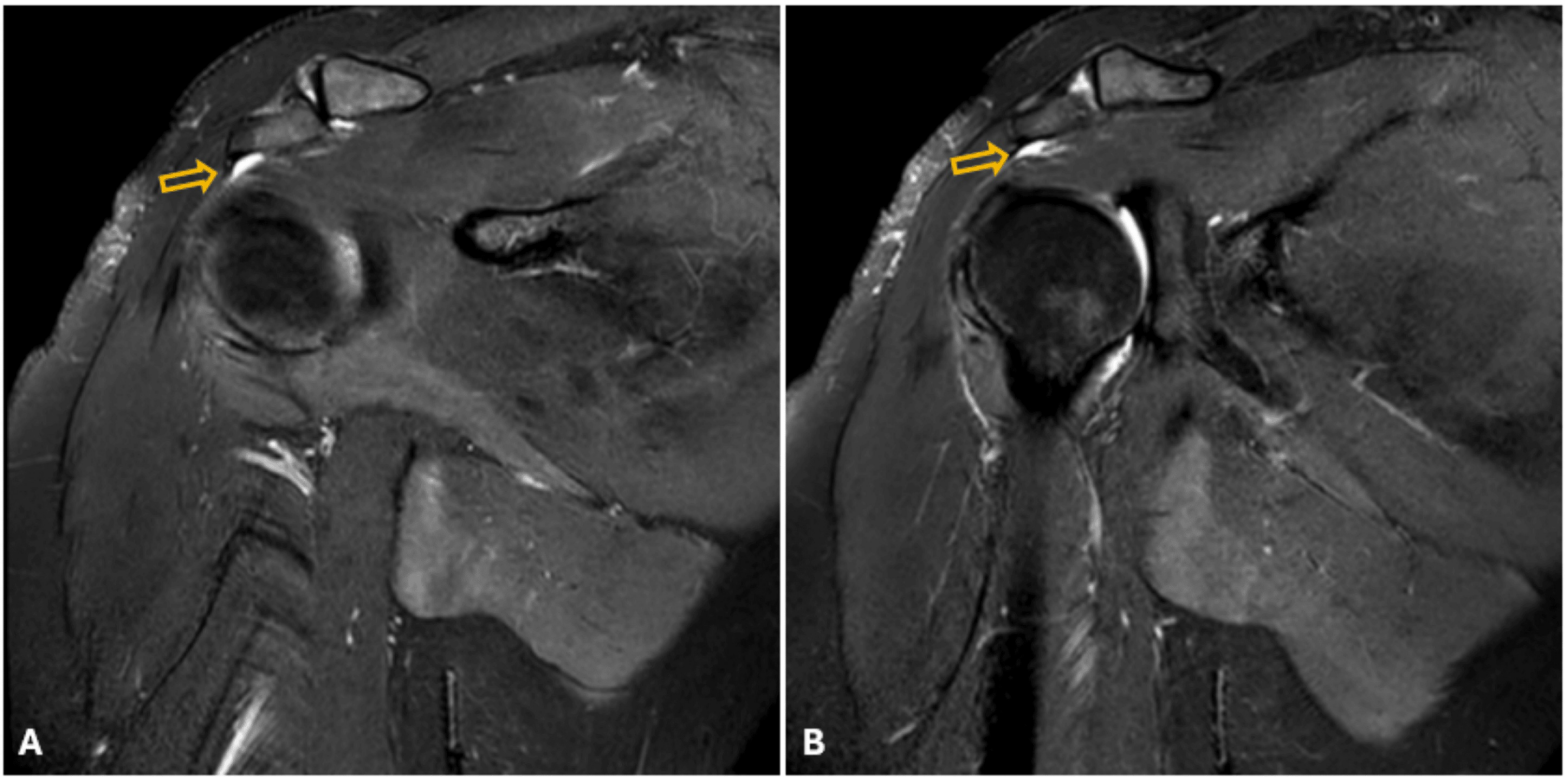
Coronal section magnetic resonance imaging (MRI) images of the right shoulder, indicating suspicion of subacromial bursitis in consecutive sequences A and B. The orange arrow shows the region of bursitis with an increased signal.
